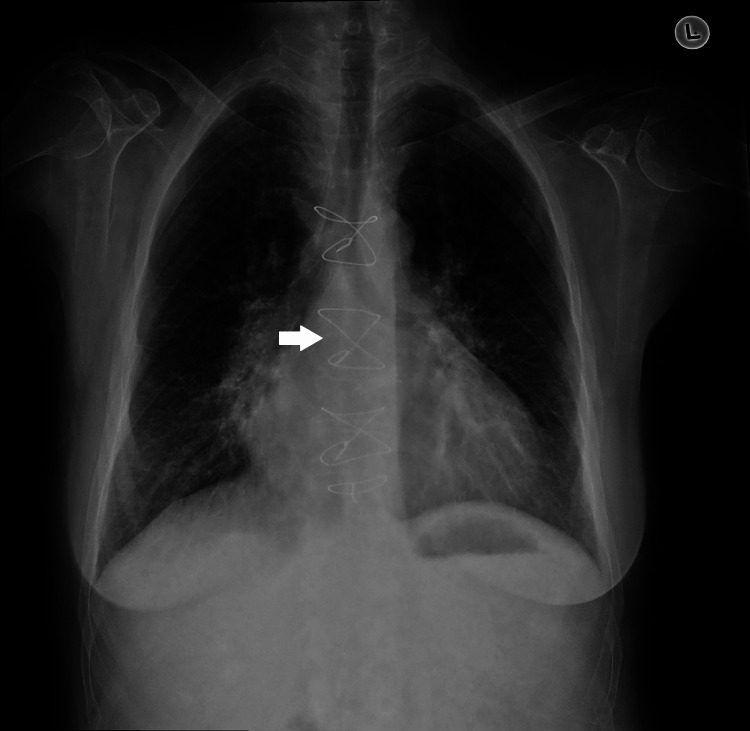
Posteroanterior chest X-ray showing cardiomegaly, clear lungs, hilar prominence, and sternal wires (indicated by a white arrow).
